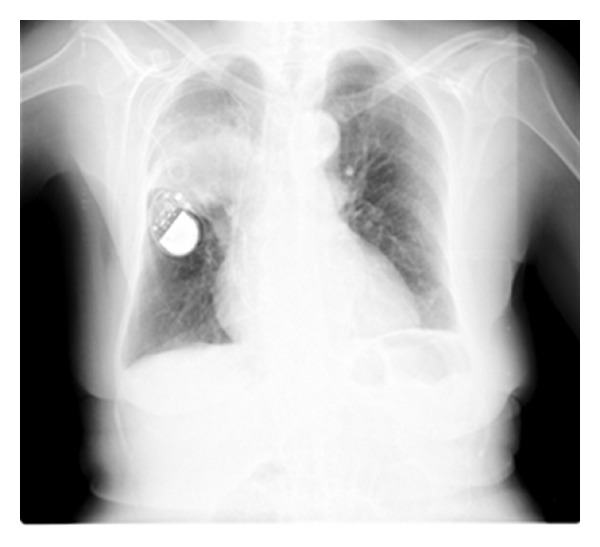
Chest X-ray revealing a heterogeneous hypotransparency in the right hemithorax upper third.
